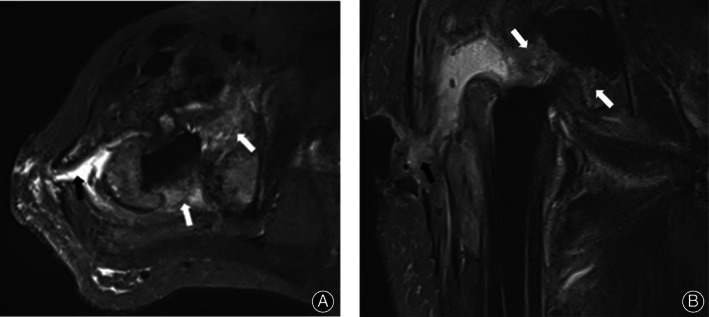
A 62‐year‐old male patient underwent THA for 1 month, and the incision healed poorly after the operation. MRI examination was performed at 1 month after surgery. T2WI‐tirm‐SEMAC axial position (A) and coronal position (B) showed edema in the joint cavity and surrounding tissues with mixed‐like high signals (white arrows) and formed sinus tracts that reached the body surface (black arrow). The patient was diagnosed with PJI in parallel with catheter irrigation and subsequent antibiotic treatment. Due to the mixed characteristics of the patient's synovium and surrounding soft tissues, both radiologists interpreted it as negative for LHS.
